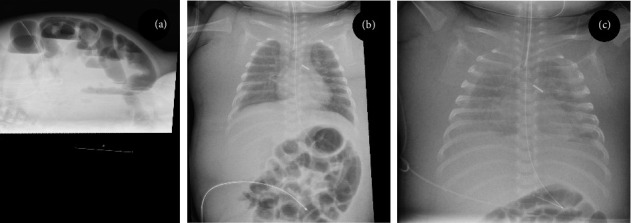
Abdominal X-ray of Patient A showing signs of bowel obstruction (a). On Day 8 after the operation, there is remarkable subcutaneous edema. Note the catheter in the left subclavian vein. Also, note the absence of edema in bowel loops (b). Despite intensive diuretic treatment edema persisted, and eventually pleural effusion developed (c).
